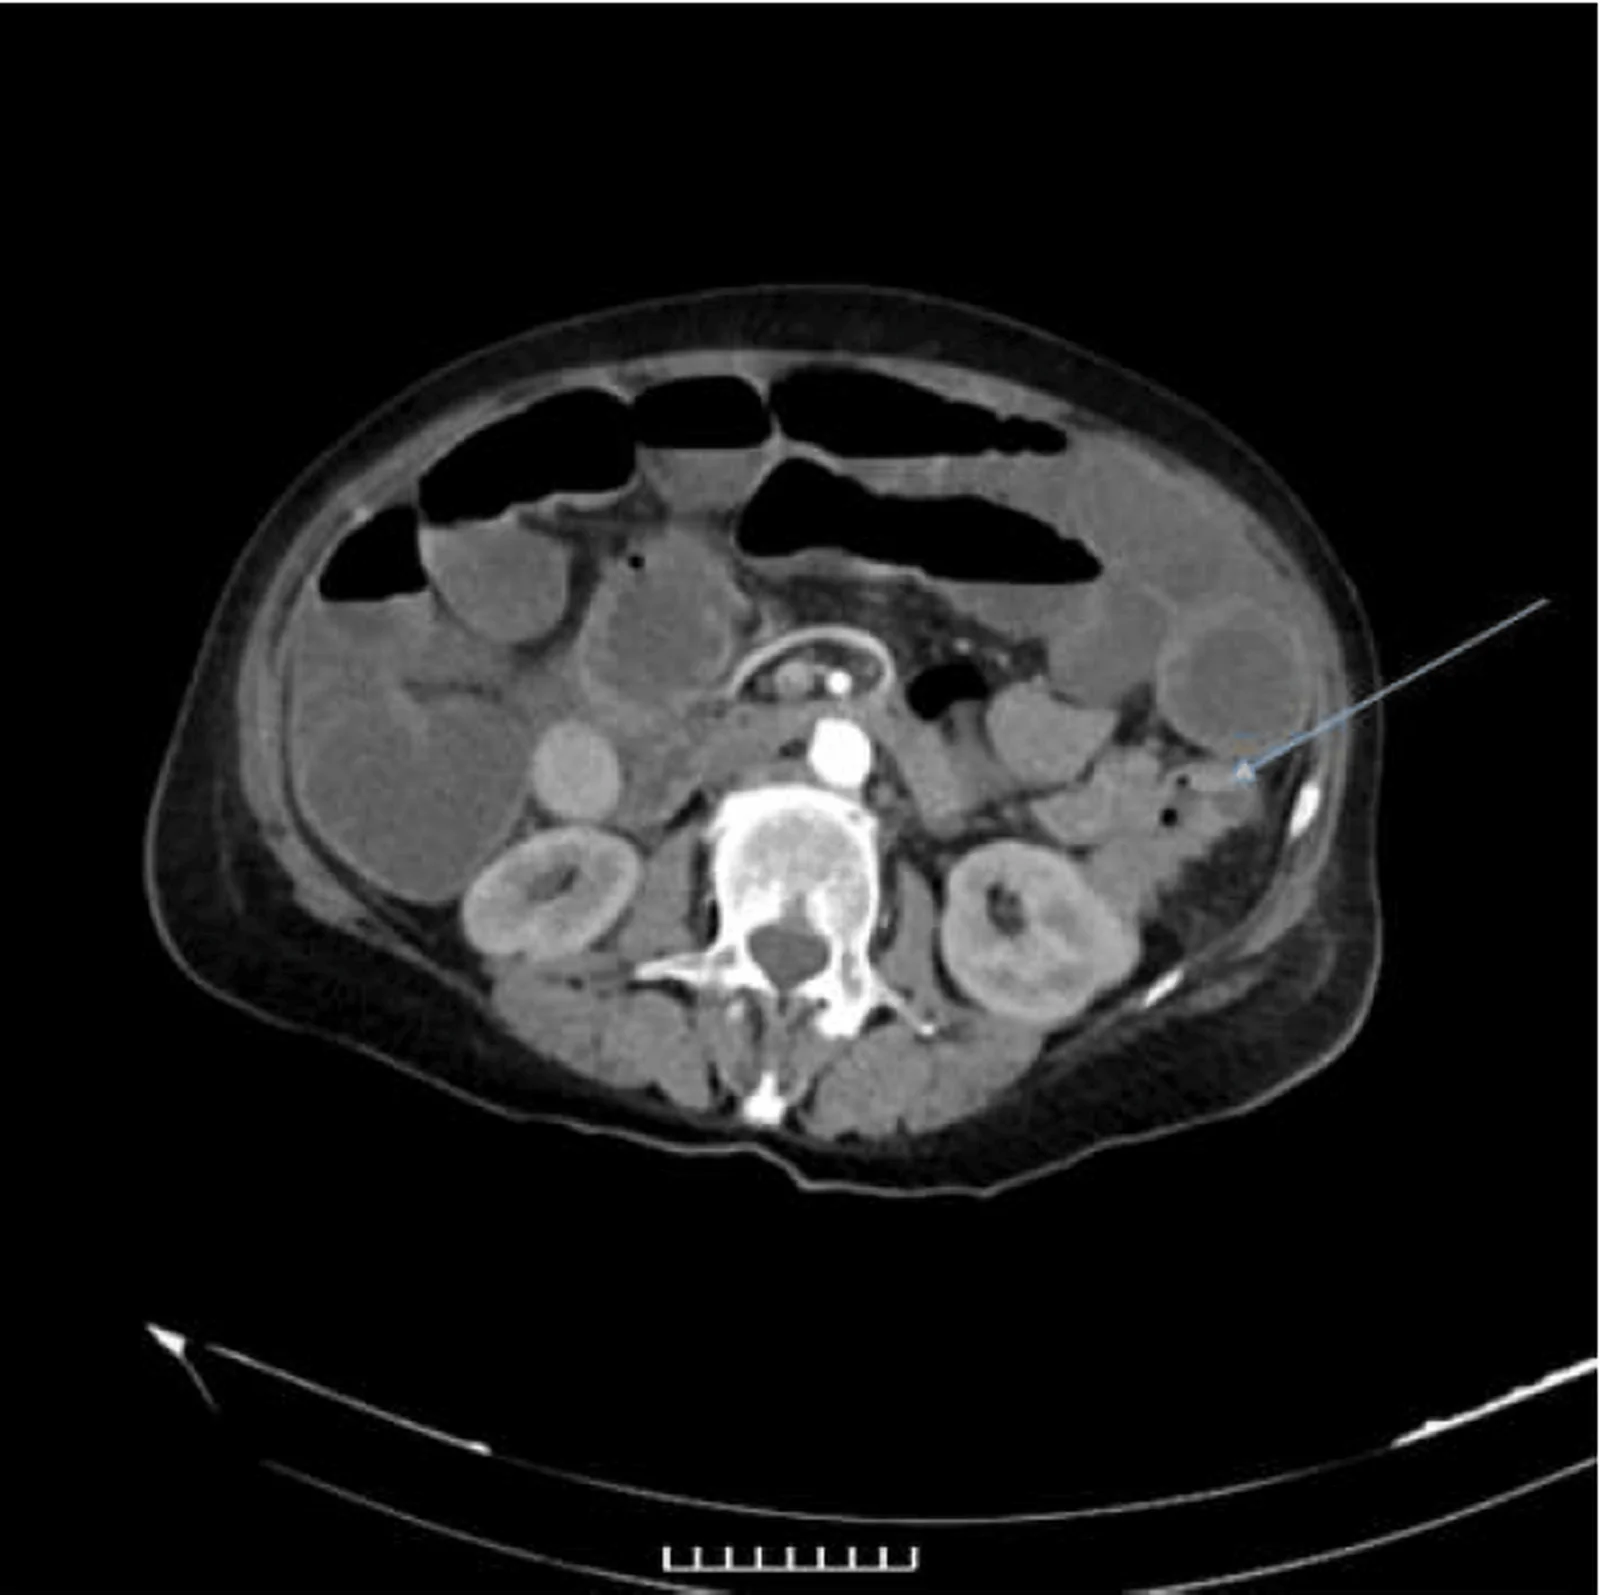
Axial abdominal CT image demonstrating a descending colon mass with retroperitoneal extension and suspected direct contact with the left kidney (arrow)
